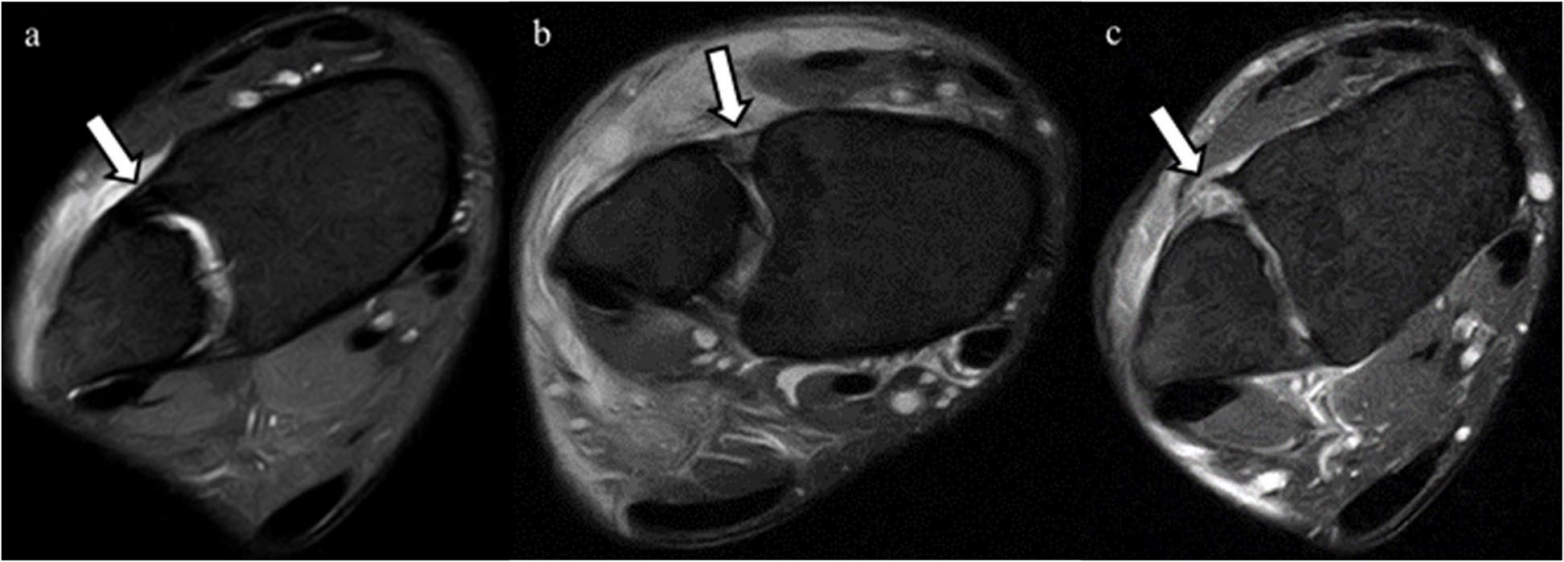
Anterior inferior tibiofibular ligament (AITFL) in oblique axial proton-density fat saturation MRI. The arrows in the figures indicate the AITFL. a The intact AITFL. b Partial tear of the AITFL, with hyperintensity of the ligament. c Complete tear of the AITFL, showing complete discontinuity
